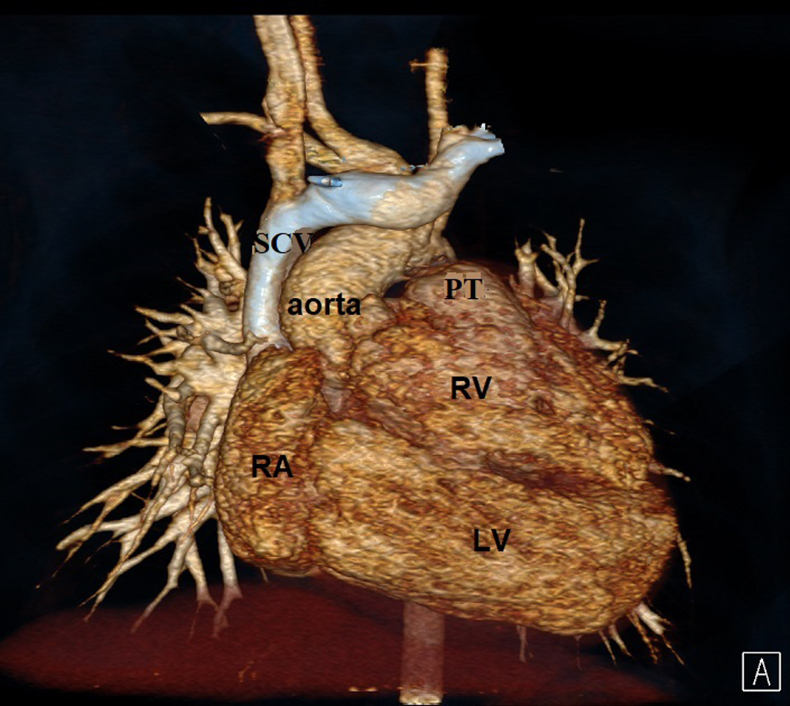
CT 3D reconstruction demonstrating the anatomy of the superior inferior ventricles.
